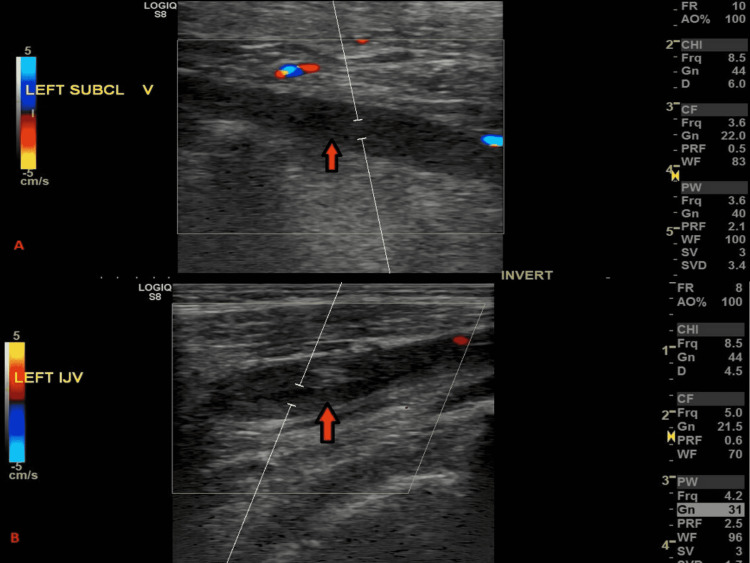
Venous duplex ultrasound showing clots (arrows) in the left subclavian vein (A) and the left jugular vein (B).
